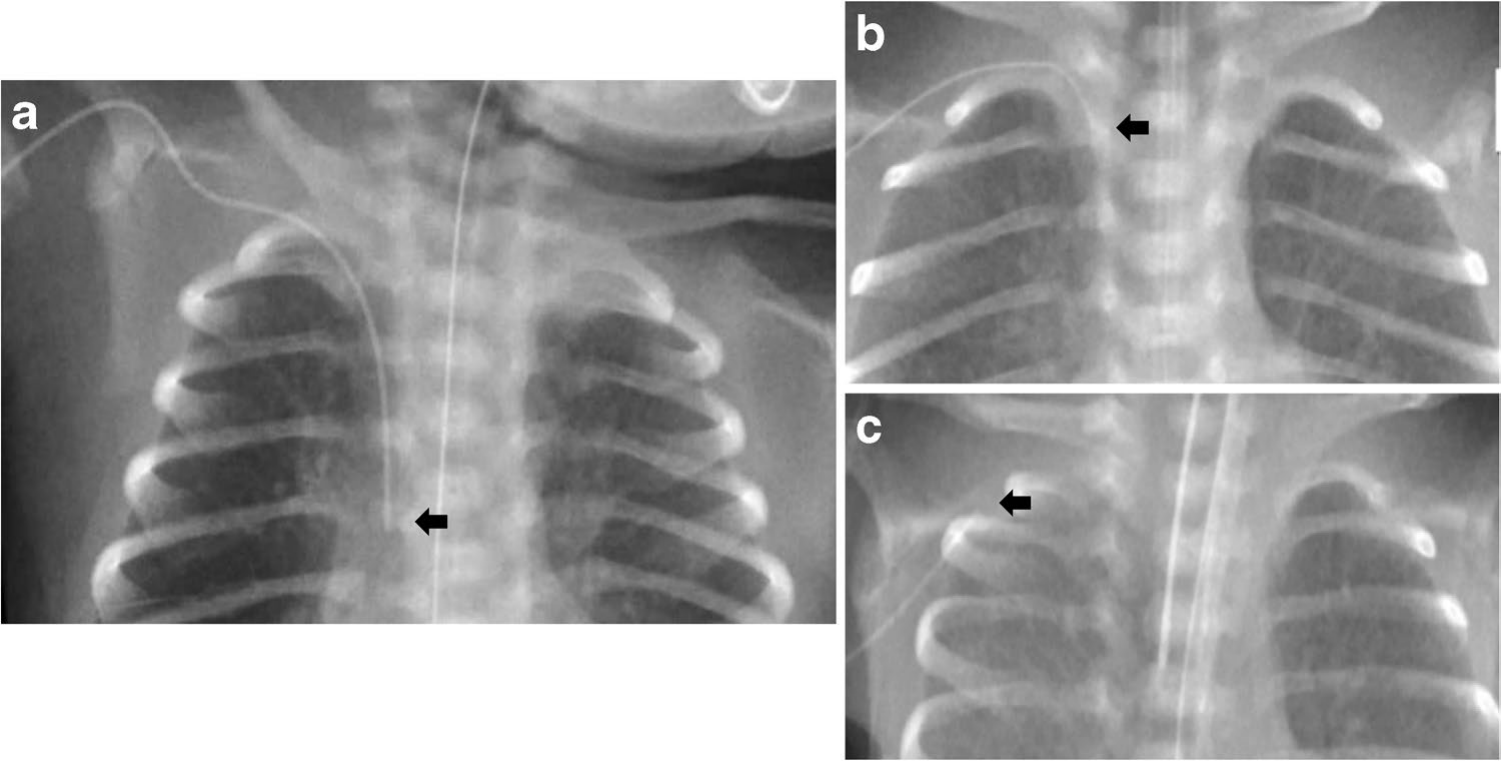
Position of peripherally inserted central catheter (PICC) tips in the upper limb. a A 5-day-old girl. Anteroposterior (AP) chest radiograph shows a PICC tip in a central ideal position—catheter tip between the 3rd and the 5th thoracic vertebrae, which corresponds to the superior vena cava and the cava-atrial junction (*arrow*). b A 1-week-old girl. AP chest radiograph shows a PICC tip in a central accept position (*arrow*): catheter tip between the medial third of the clavicle and 2nd thoracic vertebra (subclavian or brachiocephalic vein). c A 3-week-old boy. AP chest radiograph shows a PICC tip beyond the medial third of the clavicle (*arrow*)
